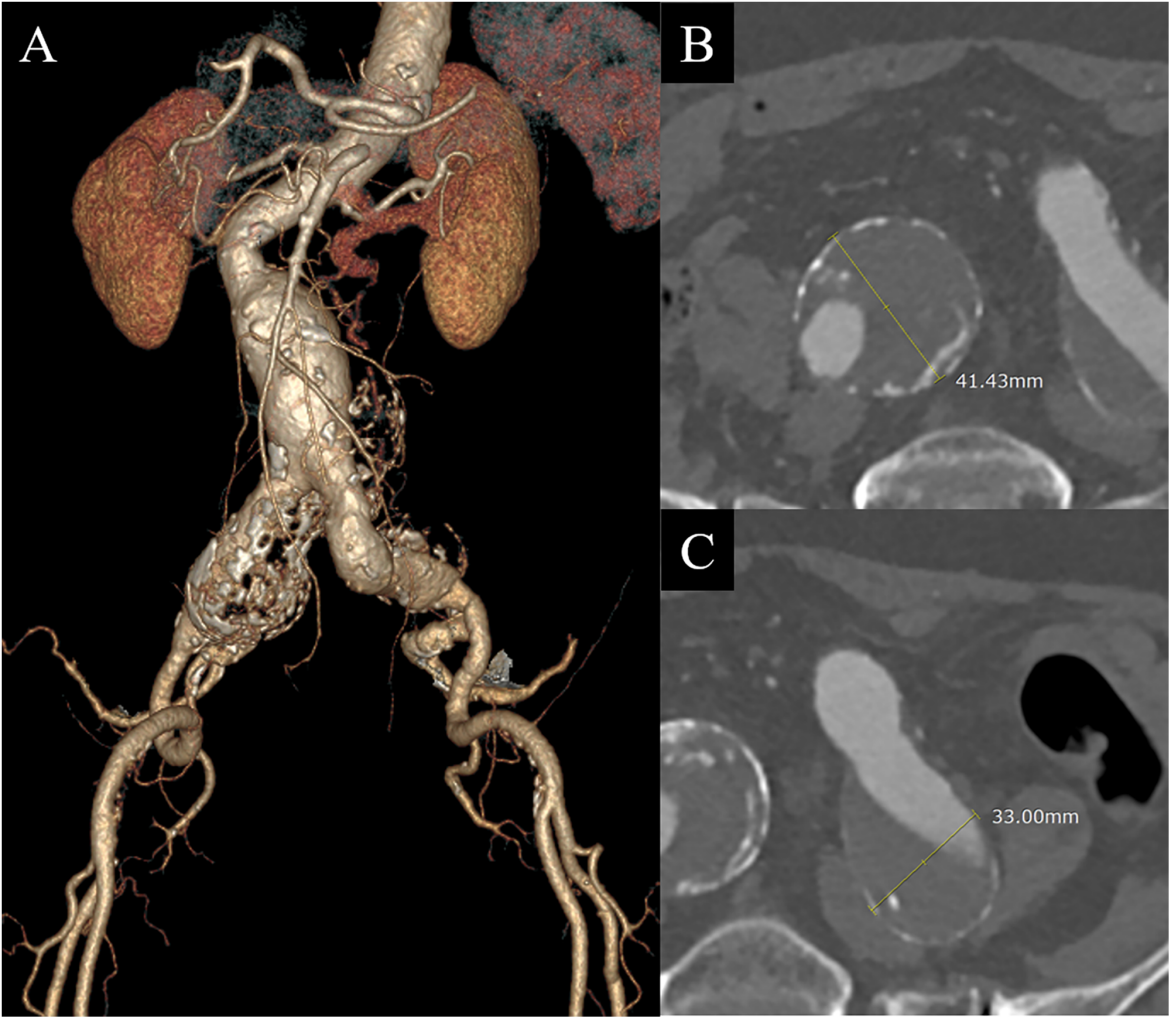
Initial computed tomography angiography (CTA) before endovascular aneurysm repair revealed a small abdominal aortic aneurysm and bilateral common iliac artery aneurysms (CIAAs). (A) 3-dimensional reconstruction image. (B) Axial image of the right CIAA demonstrating a 41 mm diameter aneurysm. (C) Axial image of the left CIAA showing a 33 mm aneurysm.
